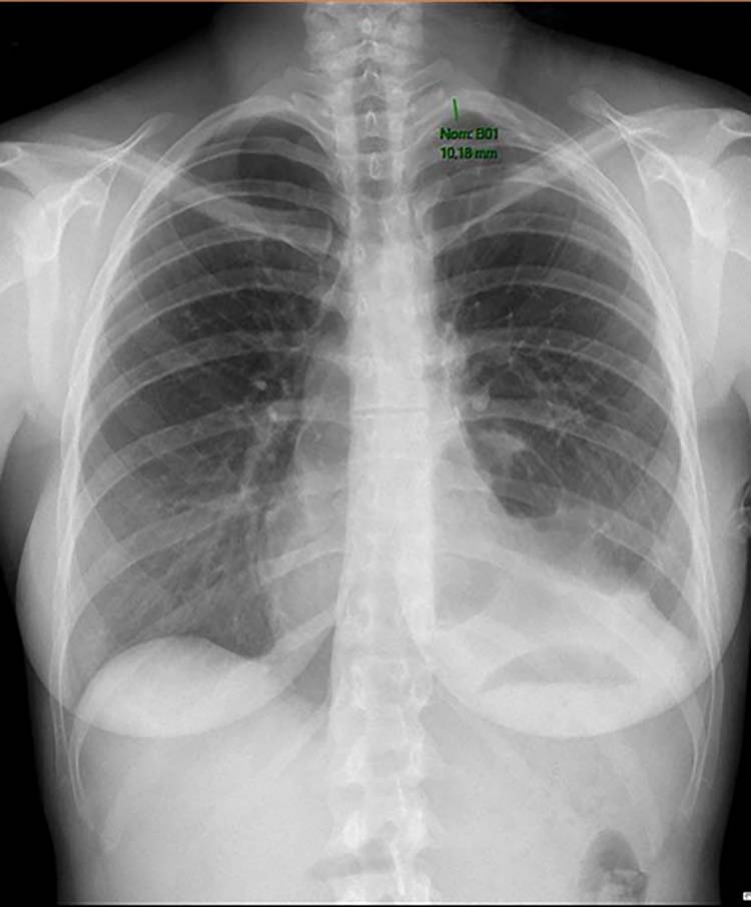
Chest radiography showing small residual pneumothorax after chest tube removal.
